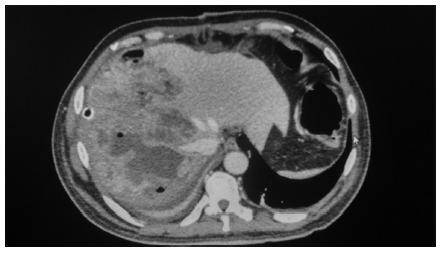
Abdominal CT showing large solid-cystic multiloculate septate liver
